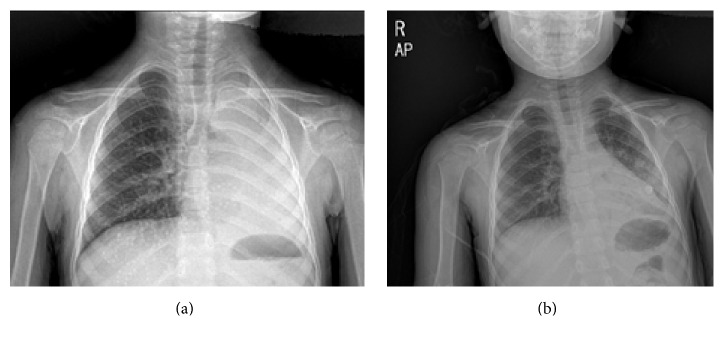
(a) Chest radiograph at admission showing complete collapse of the left lung with mediastinal shift to the left. (b) Chest radiograph showing almost complete expansion of the left lung after bronchoscopy and removal of the foreign body.
